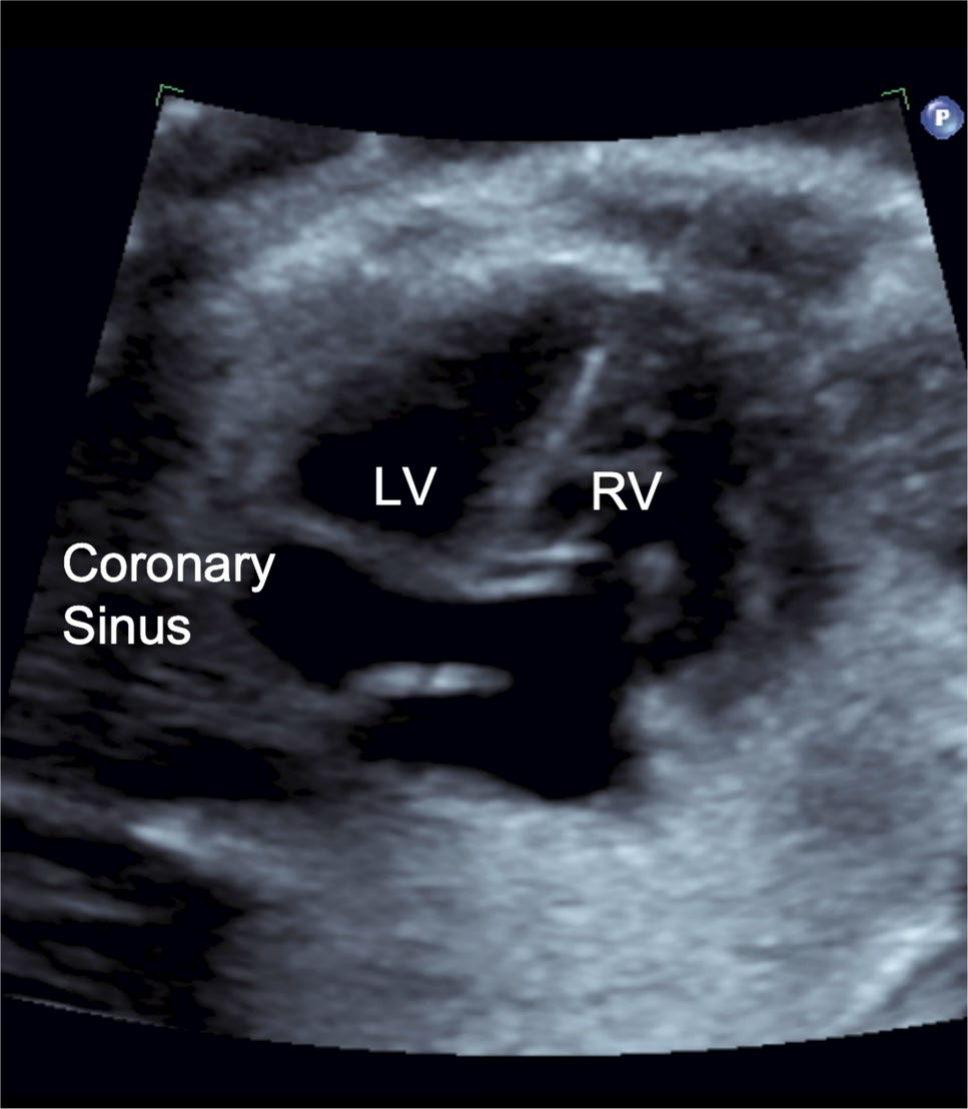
Image from a fetal echocardiogram showing a dilated coronary sinus a common finding in PLSVC
